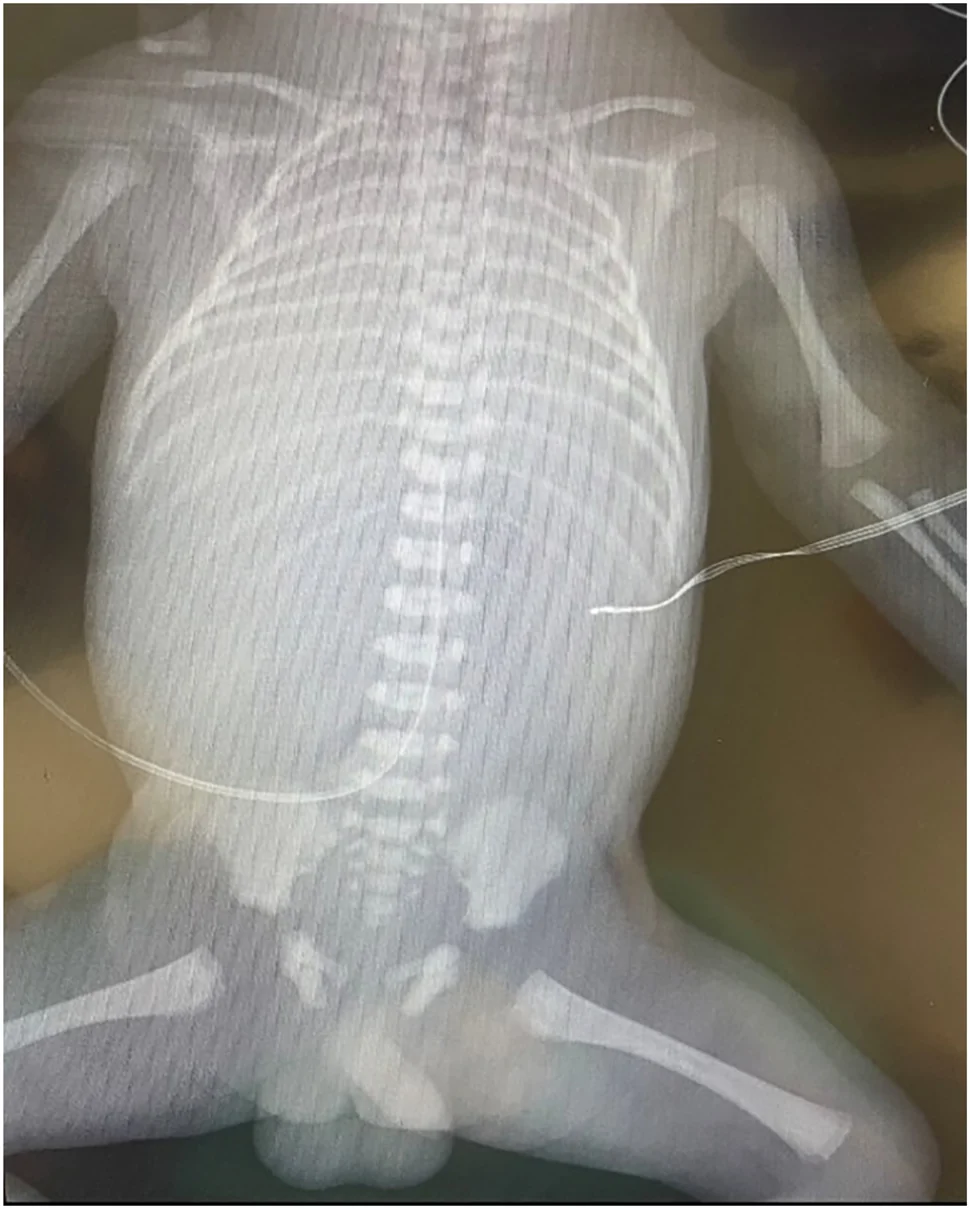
X-ray showing complete opacification of lungs and abdomen, small chest and dilated esophagus.
